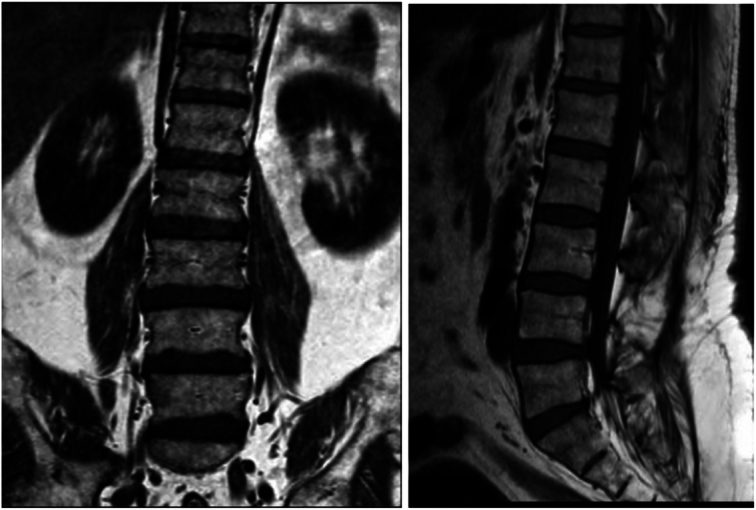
MRI lumbar spine with and without IV contrast: slight enhancement of the cauda equina can be seen along with arachnoiditis.
